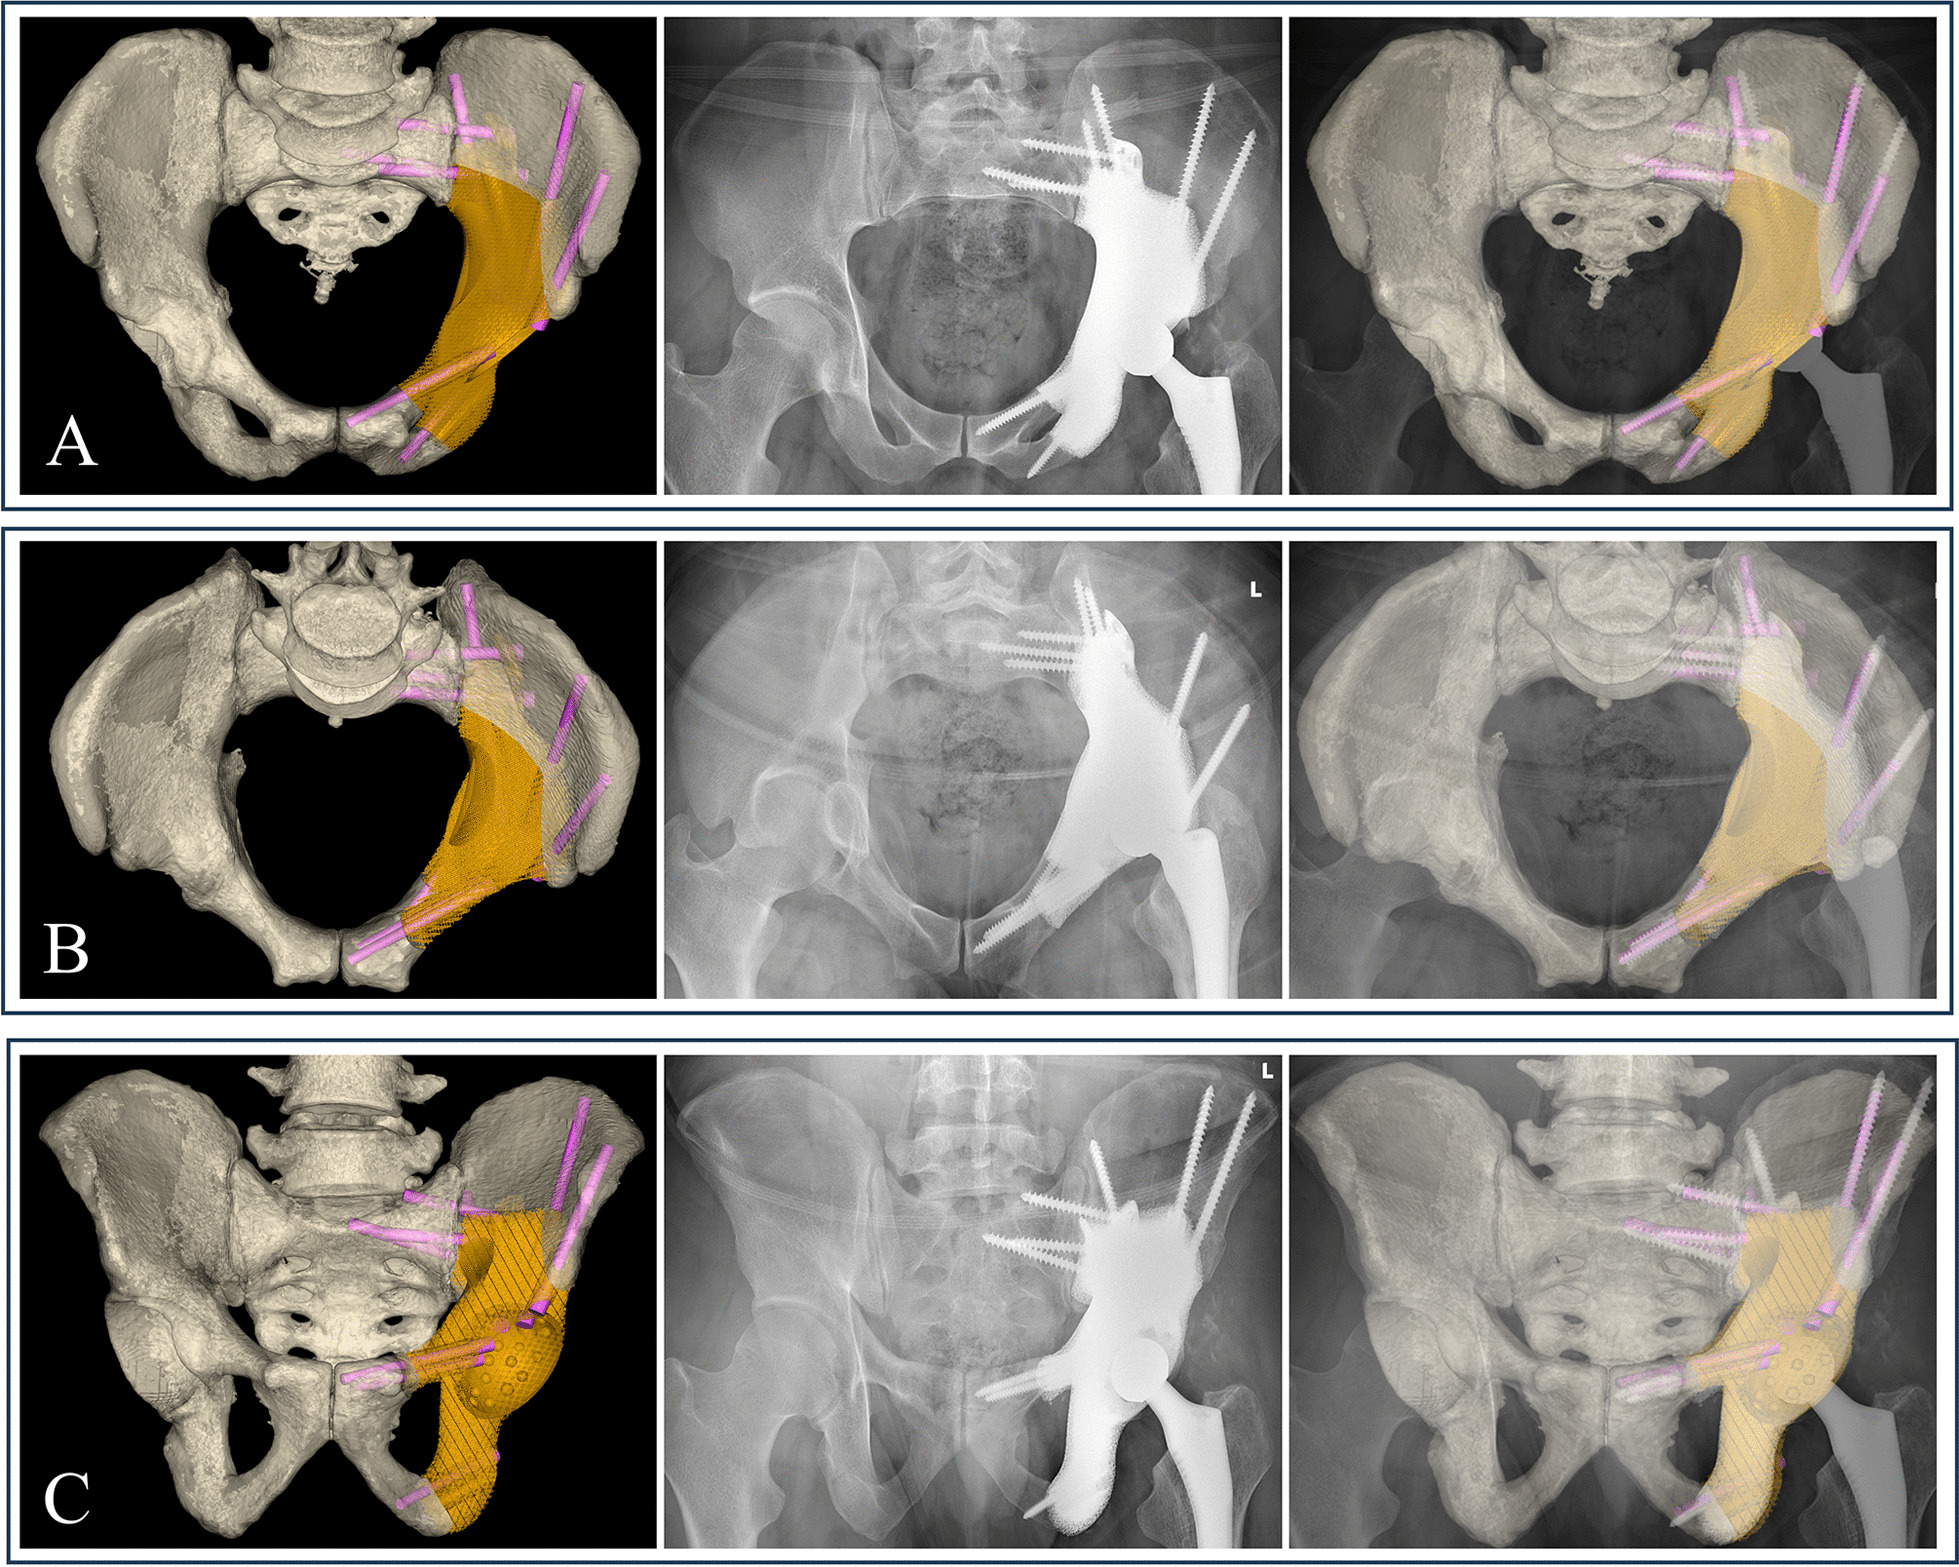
Accuracy evaluation. Postoperative anteroposterior A inlet, B and outlet C pelvic radiograph demonstrated precise reconstruction
